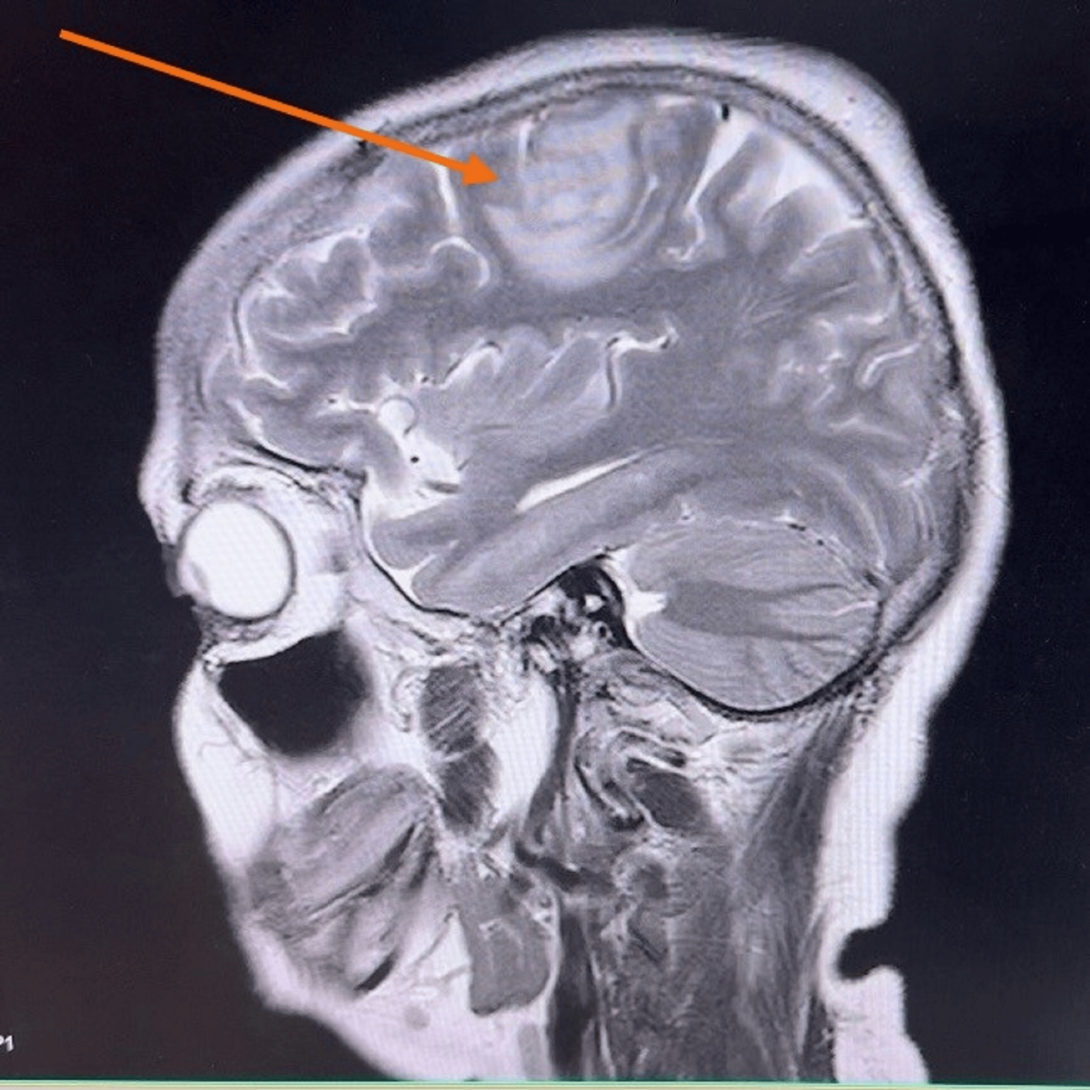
Coronal section of the patient’s brain contrast MRI showing brain abscess in left frontal lobe (24 mm × 15 mm × 17 mm) (red arrow) and a further small area of early abscess formation in the right posterior temporal lobe.
